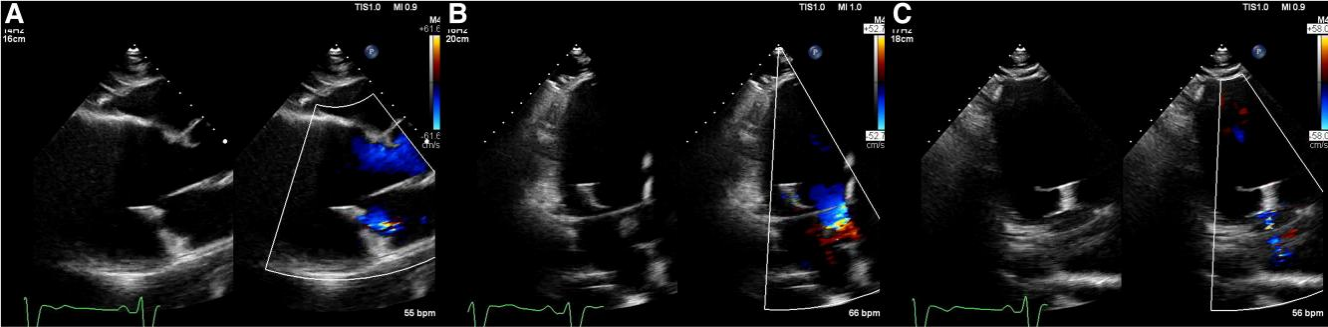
Postprocedural transthoracic echocardiography images showed mild residual mitral regurgitation (*A–C*).
